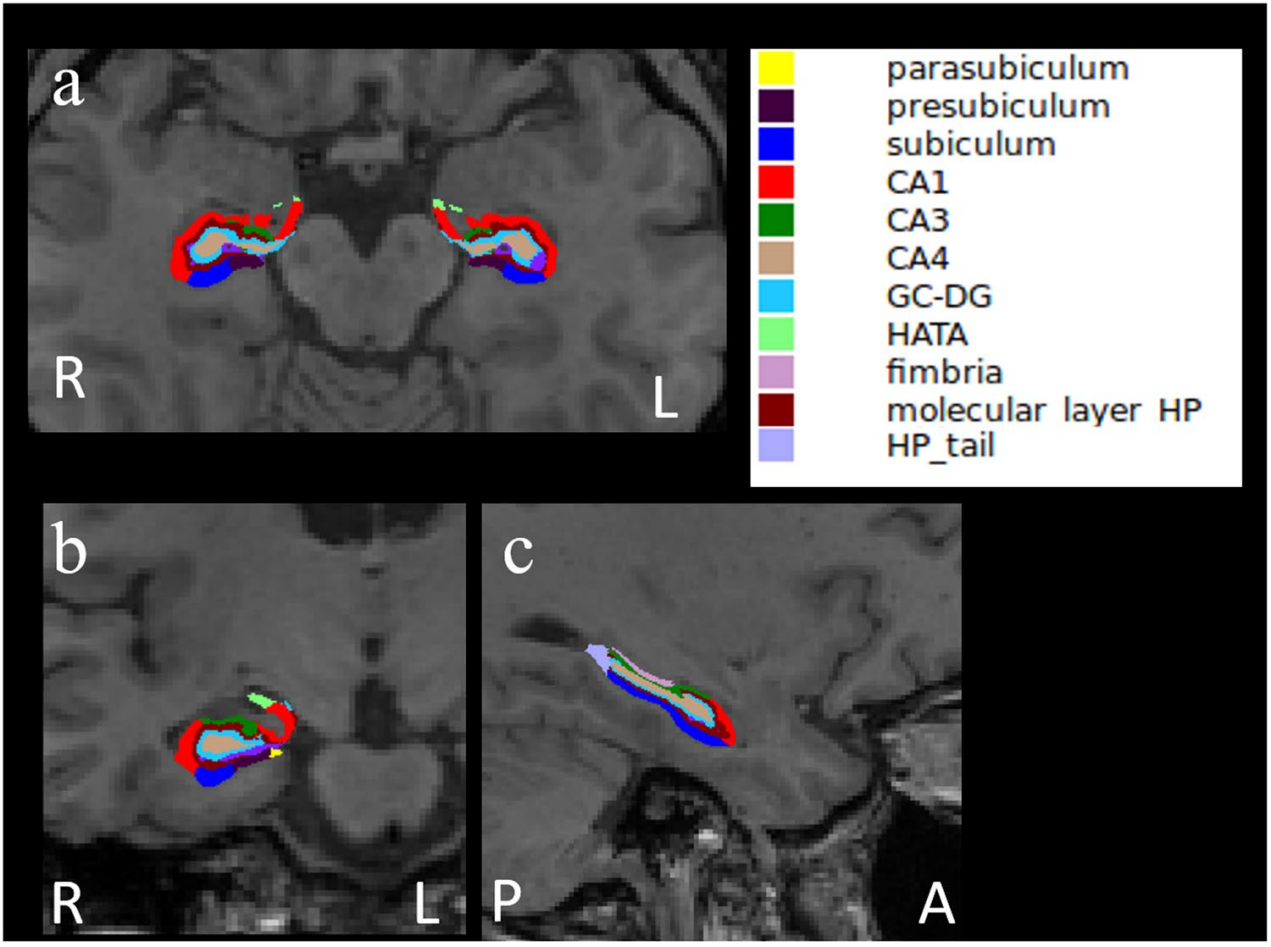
The representative subdivision of the hippocampal subfields. The mask of each region overlapped on the axial (a), coronal (b), and sagittal (c) T1-weighted images. R, right; L, left; A, anterior; P, posterior. Color classification: parasubiculum, yellow; presubiculum, dark purple; subiculum, blue; CA1, red; CA3, dark green; CA4, brown; granule cell layer of dentate gyrus (GC-DG), sky blue; hippocampus-amygdala-transition-area (HATA), green; fimbria, purple; molecular layer hippocampus (HP), dark brown; hippocampal tail, gray.
